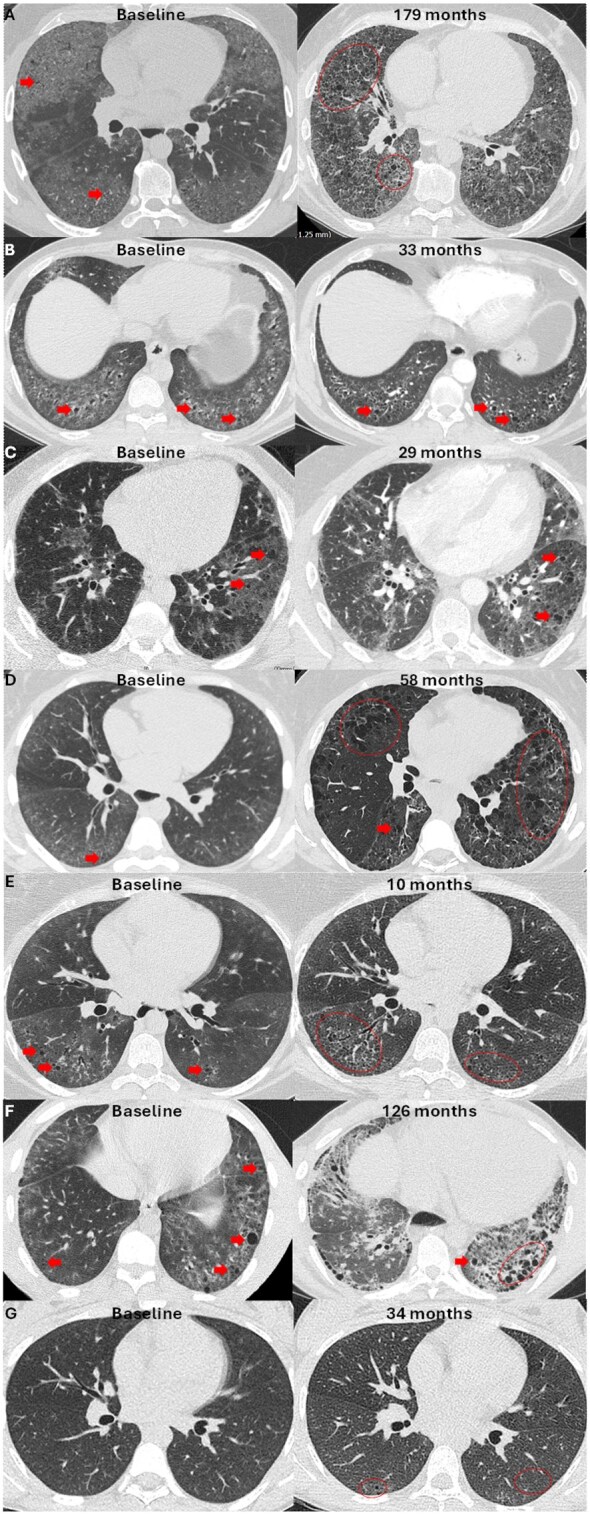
CT images at baseline/diagnosis with SARD (left) and follow-up (right). (A–F) Baseline images show ground glass opacification typical of cellular NSIP pattern lung disease with early internal cystic destruction evident within areas of ground glass. (E) Minor reticular changes within the ground glass and incidental pneumomediastinum. (G) The absence of ILD at presentation. Follow-up images show varying degrees of progression with more conspicuous destructive cystic changes most evident in A–C. Cystic destruction is more subtle in E and G. Background established fibrosis is evident in A and F at follow-up yet is discrete from cystic destruction. All baseline images were performed <6 months after diagnosis with SARD. Unannotated images are available (Supplementary Fig. S3).
